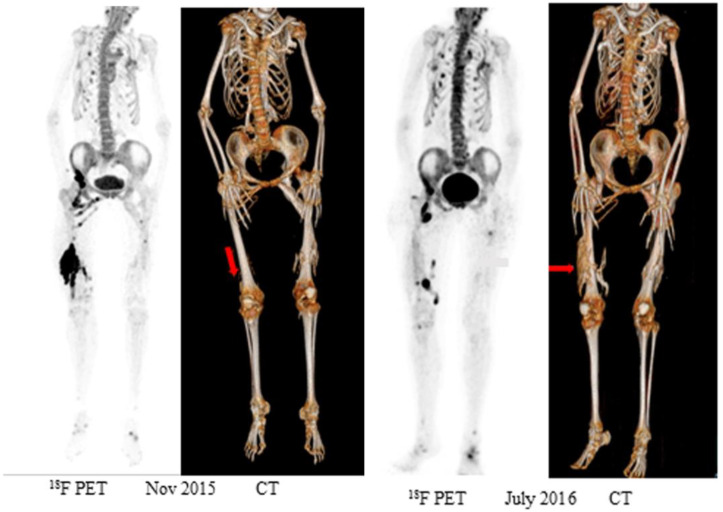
Whole-body [^18^F]NaF PET maximum intensity projection (MIP) (left panel) and corresponding low-dose CT 3D reconstruction (right panel) of a patient with FOP (Nov. 2015) and consecutive scan (July 2016). [^18^F]NaF tracer uptake is displayed on a grey scale, with black indicating a higher tracer accumulation. [^18^F]NaF PET shows initially a lesion with increased tracer uptake in the upper right leg in the quadriceps muscle, while the corresponding CT shows no apparent HO. Eight months later, the increased tracer uptake in the right quadriceps muscle subsided, while the low-dose CT shows a large HO lesion, analogous to the site of increased tracer uptake in Nov. 2015. (Reprinted from Bone, Vol. 109, Eekhoff EMW, Botman E, Coen Netelenbos J, et al., [^18^F]NaF PET/CT scan as an early marker of heterotopic ossification in fibrodysplasia ossificans progressiva, 143–146, Copyright (2018), with permission from Elsevier.).
